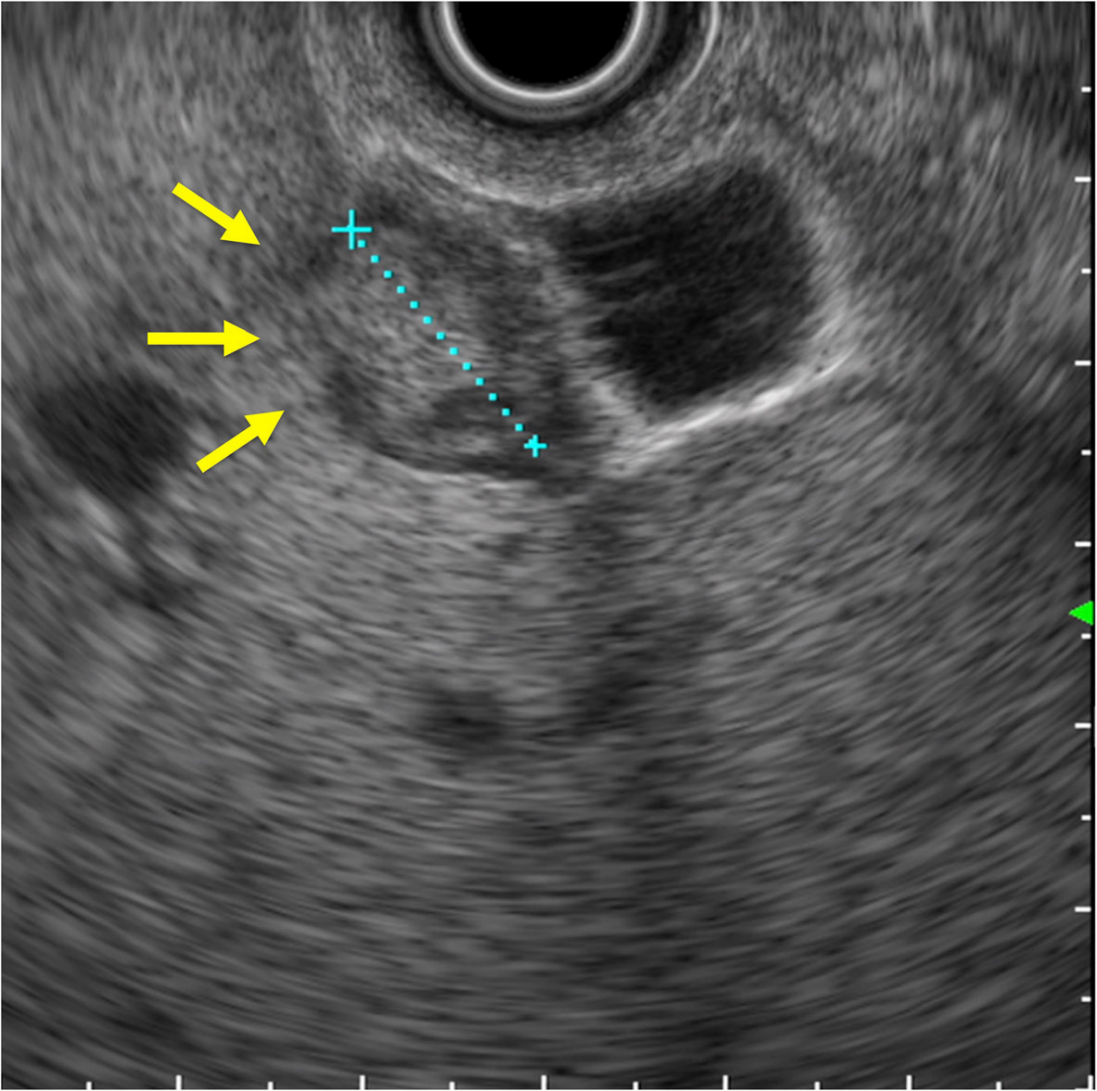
EUS imaging. Endoscopic ultrasonography demonstrated a solid tumor in the proximal gallbladder that seemed to invade the hepatic parenchyma adjacent to the gallbladder (see the arrows). The dotted line indicates the size of the mass
